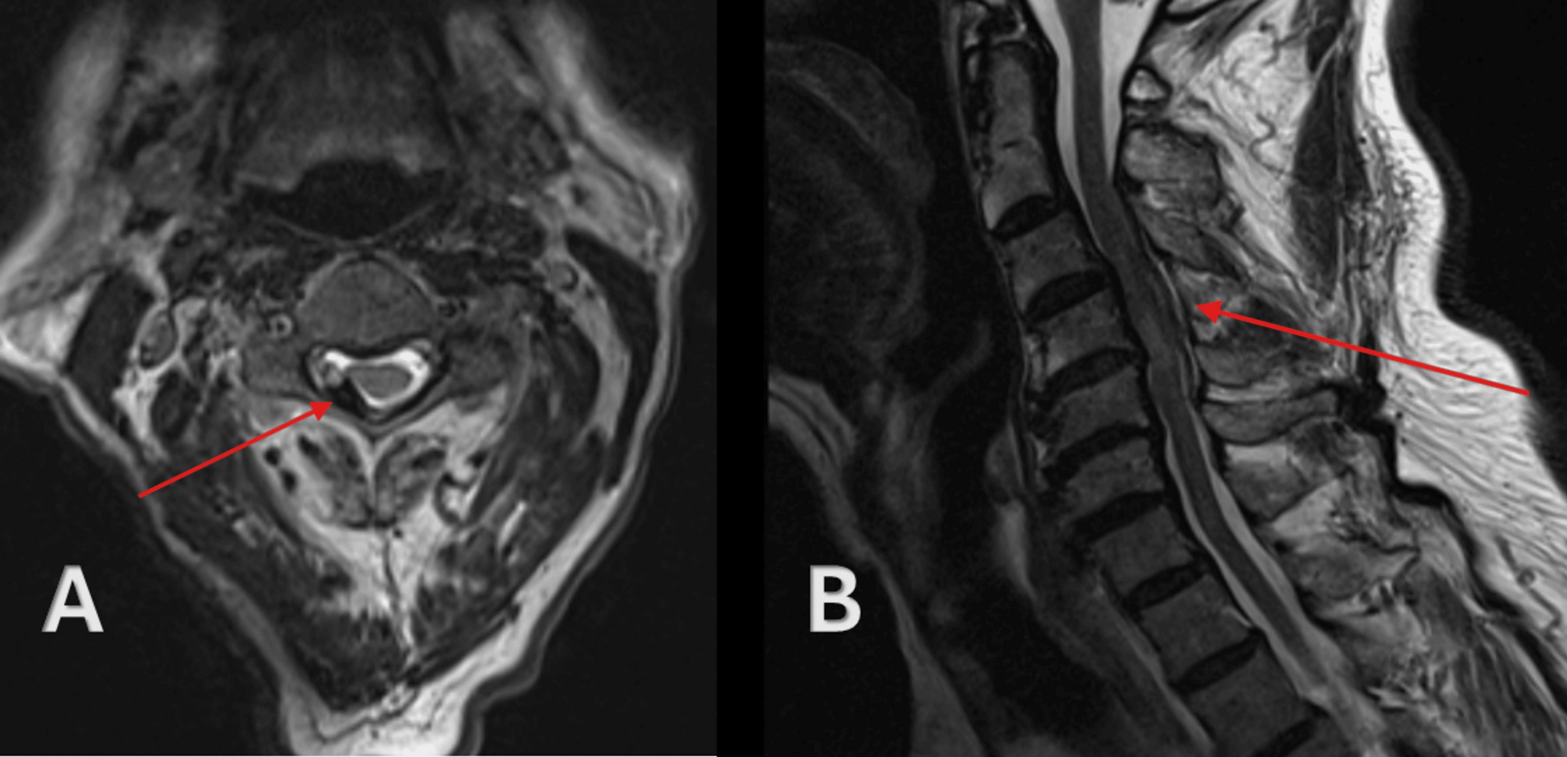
Axial T2-weighted MRI of the cervical spine (A) showing a spinal epidural hematoma, and sagittal T2-weighted MRI (B) demonstrating cervical spinal cord compression with associated myelopathic signal changes. MRI, magnetic resonance imaging
